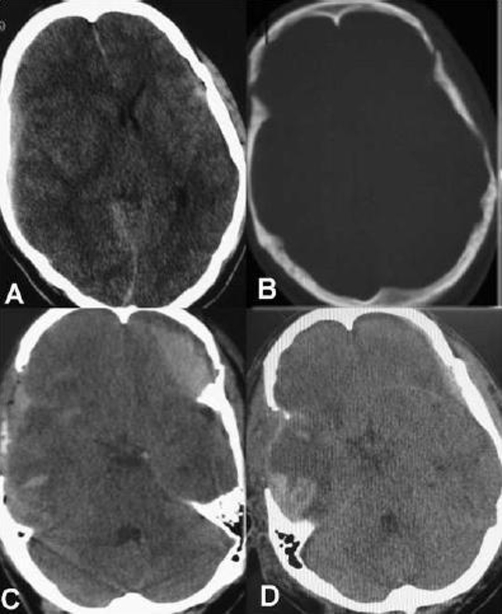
Preoperative axial brain CT, showing right acute subdural hematoma with midline shift and left small EDH (A); axial bone window showing linear skull fracture adjacent to the EDH site (B); post-operative brain CT scan showing expansion of the EDH after decompressive surgery for evacuation of acute subdural hematoma (C); delayed CT scan showing spontaneous resolution of the EDH after 5 days (D).
